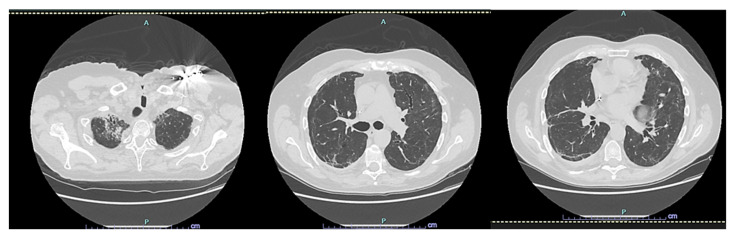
CT scan HRCT of the patient later, at 24 months.
